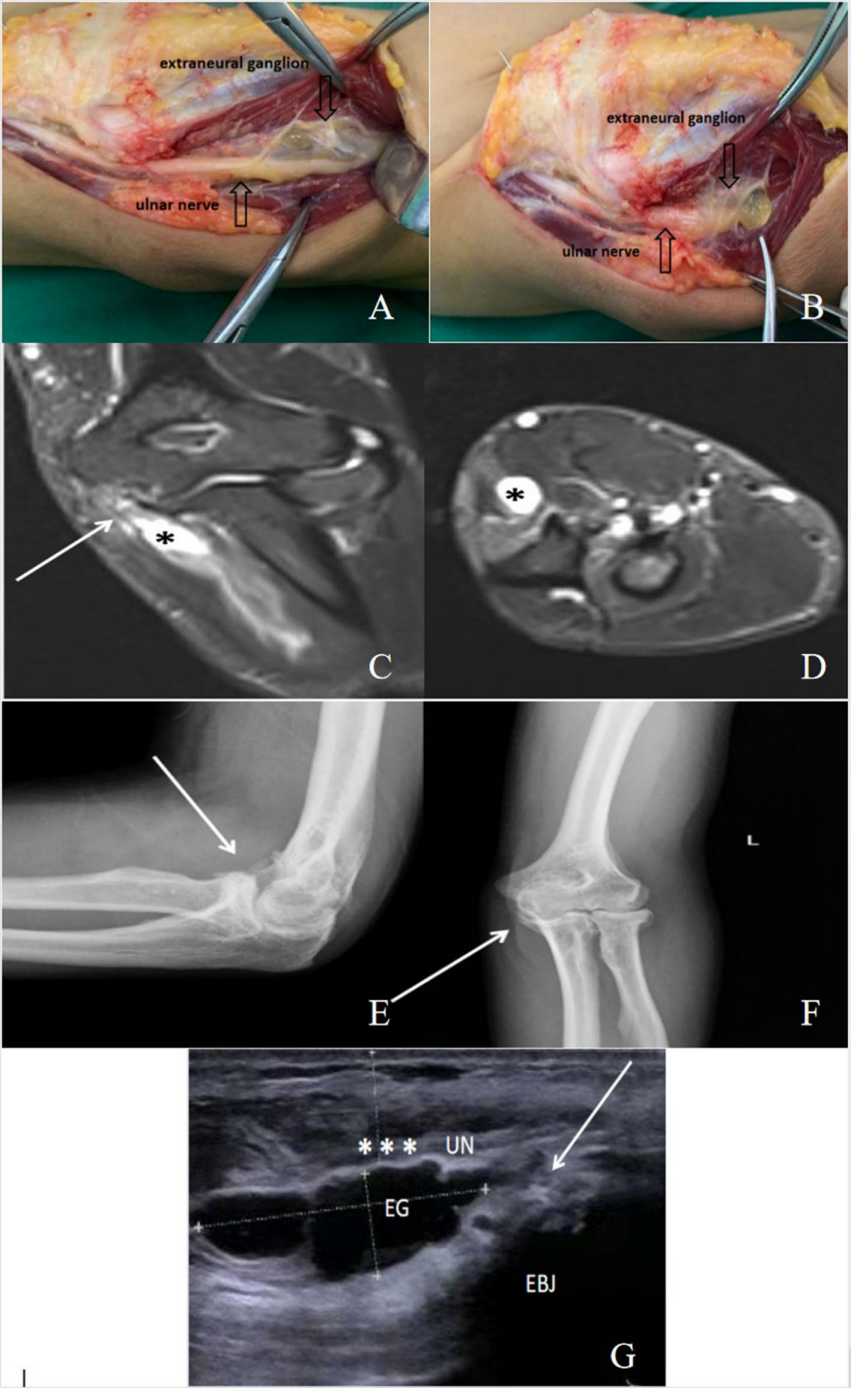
A 50-year-old male patient with cubital tunnel syndrome caused by extraneural ganglion (EG). (A,B) Intraoperative photograph of the extraneural ganglion, which is compressing and flattening the ulnar nerve in the cubital tunnel. (C,D) The extraneural ganglion (*) is shown as (C), a high signal in T2STIR in sagittal view, and (D) a high signal in T2STIR in transverse view near the elbow joint. (E,F) Anteroposterior and lateral X-ray films of the elbow joint, showing the existence of elbow arthritis (arrow). (G) An ultrasound image showing an extraneural ganglion (EG) compressing the ulnar nerve (UN*). EBJ: elbow joint.
